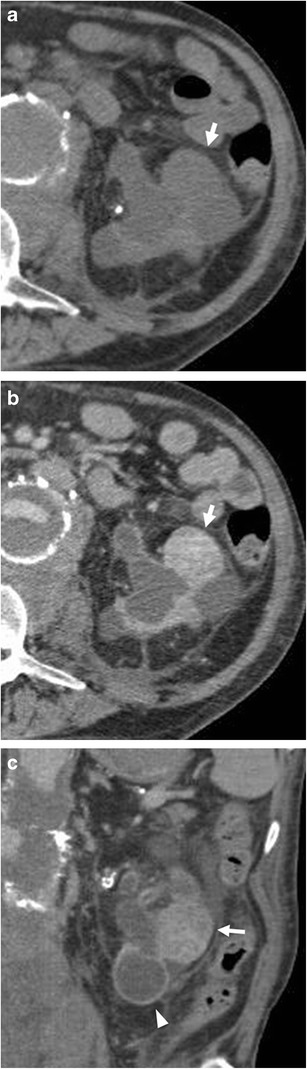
a–c: ACKD in a patient who has been undergoing haemodialysis for 10 years. a Unenhanced and contrast-enhanced CT, transverse plane (b) and coronal reformation (c). Both kidneys present multiple cysts; one of these at the inferior pole of the left kidney presents uniform thickening of the wall (Bosniak IIF) (*arrowhead*). Moreover a solid enhancing lesion, about 3 cm in size, is evident on the left kidney (*arrow*) corresponding to a clear-cell renal cell carcinoma
